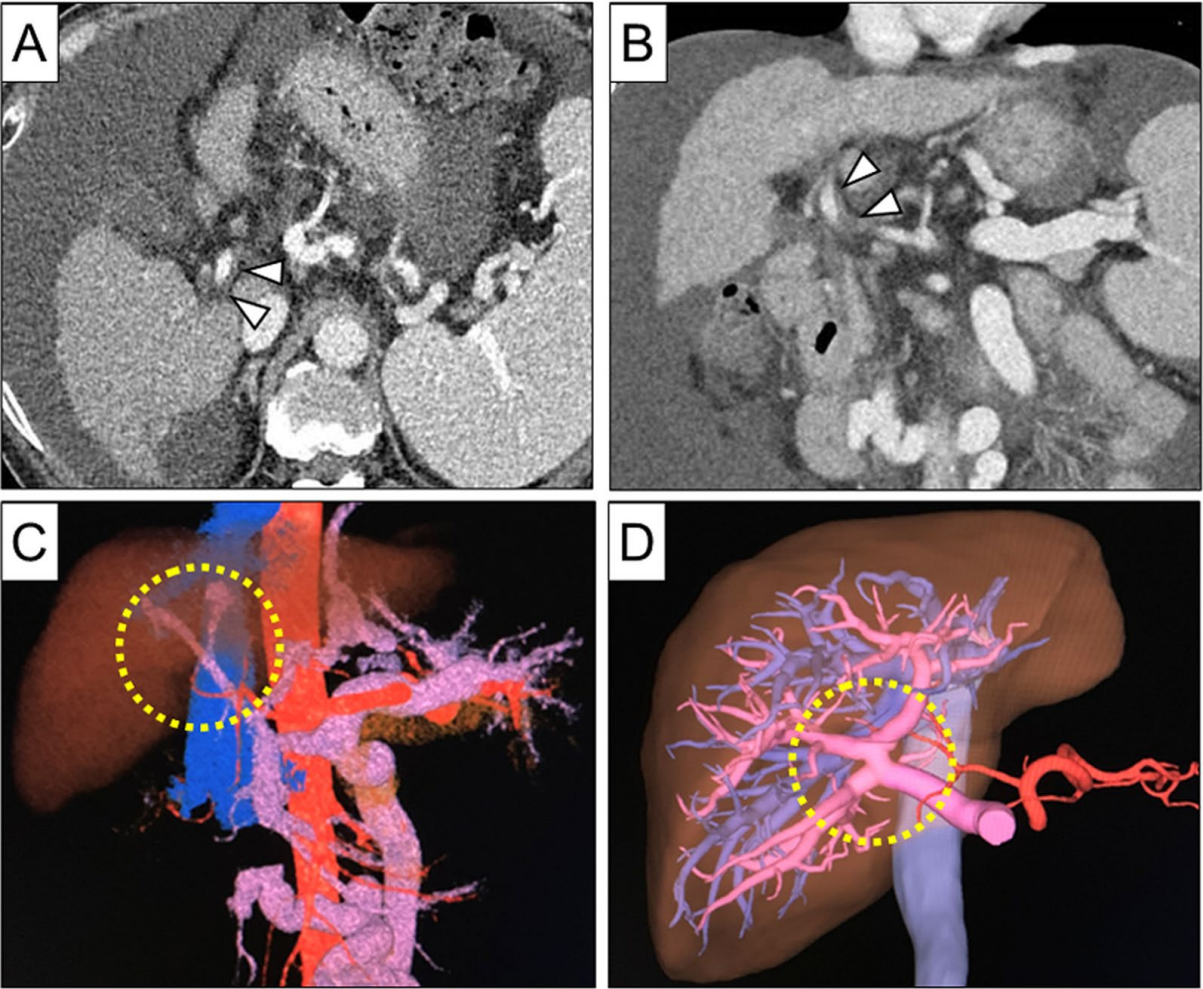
Preoperative imaging of recipient and donor. A, B Contrast-enhanced computed tomography showed a partially thrombosed main portal vein in the recipient (arrowheads). C Three-dimensional computed tomography showed the recipient’s portal vein which had standard anatomy and was narrowed by portal vein thrombosis (circle). D Three-dimensional computed tomography showed separating right anterior and right posterior portal veins in the donor (circle)
